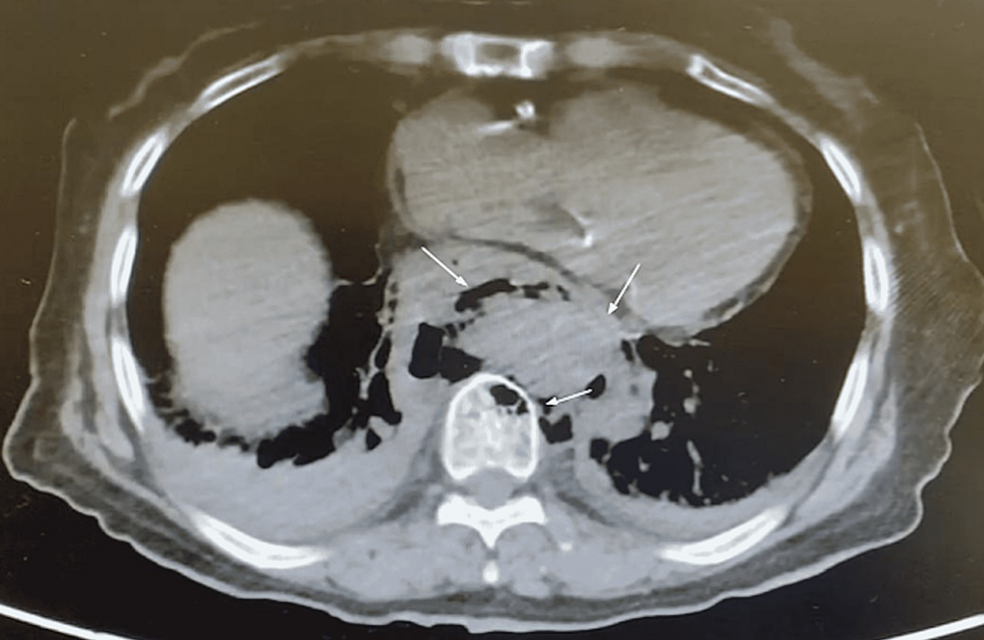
CT abdomen and pelvis without contrast. Air-fluid levels are apparent in the mediastinum as well as the vertebral body. Aortic involvement is also highlighted (arrows).
